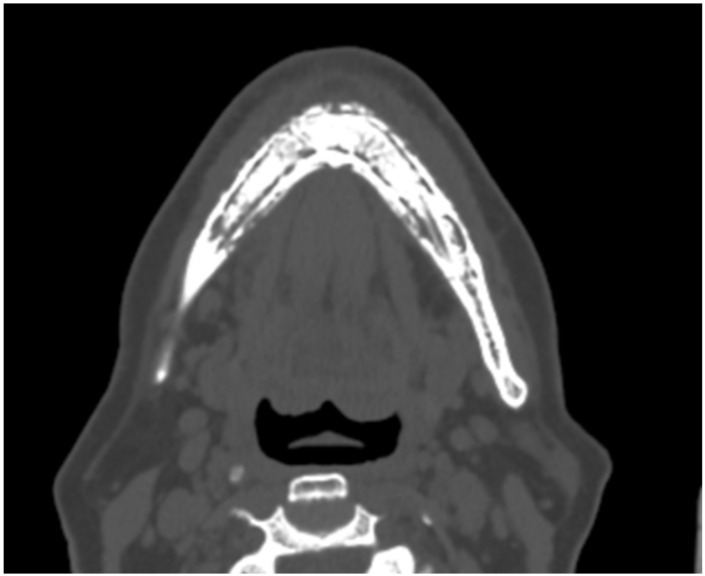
CT scan of one of our patients showing typical radiographic findings of Stage III MRONJ (full-thickness bone necrosis, diffuse periosteal reaction and sequestrum formation).
